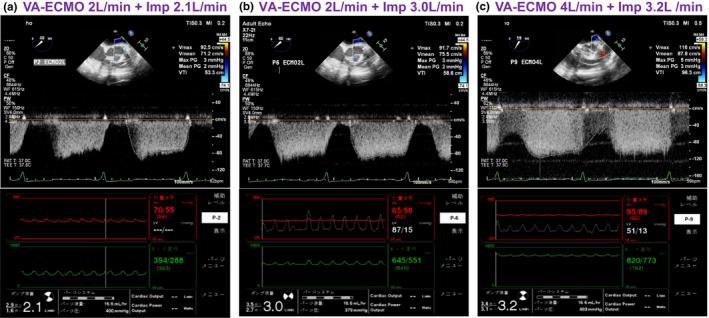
Left coronary anterior descending artery flow, the aortic pressure, and the Impella motor current waveforms on the Impella controller at different hemodynamic support and LV unloading conditions. Panel (a) shows the pulsed Doppler blood flow of the left anterior descending artery (LAD), and the corresponding aortic pressure and Impella motor current waveforms on 2.0 L/min VA‐ECMO and 2.1 L/min Impella support. Panel (b) shows LAD flow, the aortic pressure, estimated LV pressure, and Impella motor current waveforms on 2.0 L/min VA‐ECMO and 3.0 L/min Impella support. Panel (c) shows LAD flow, the aortic pressure, estimated LV pressure, and Impella motor current waveforms on 4.0 L/min VA‐ECMO and 3.2 L/min Impella support. The LAD flow was not significantly increased when blood was ejected from the LV [panels (a) and (b), partial LV support] despite mean Impella support was increased from 2.0 to 3.0 L/min. When the aortic pressure became non‐pulsatile and the estimated systolic LV pressure never reached the aortic pressure throughout the entire cardiac cycle (estimated peak aortic and the LV pressures were 95 and 51 mmHg, respectively) and the blood ejection from the LV was fully bypassed by Impella (LV uncoupling), LAD flow became continuous, and the peak flow velocity and corresponding VTI were markedly increased [panel (c)]. It is noted that the LAD internal diameter measured by the B‐mode echocardiogram was not changed at different VA‐ECMO and Impella support conditions (4.2 mm, data not shown)
